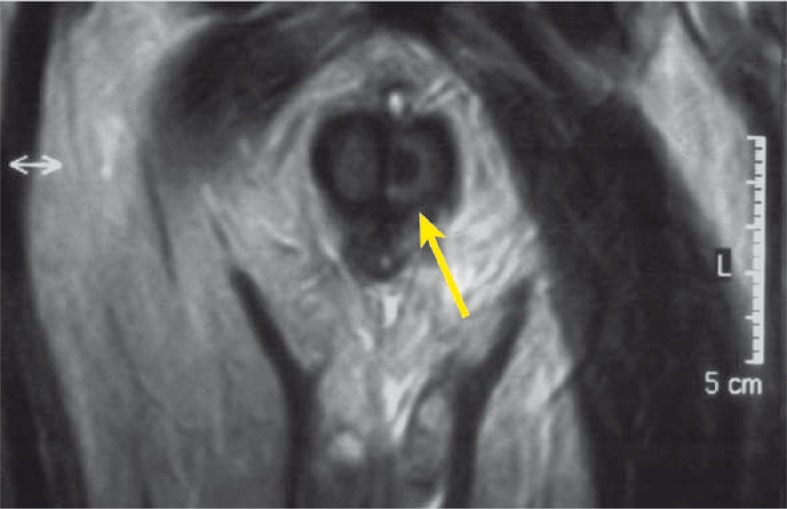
Axial T2-weighted MR image was showed nodular metastatic involvement of the penis shaft (arrow).
